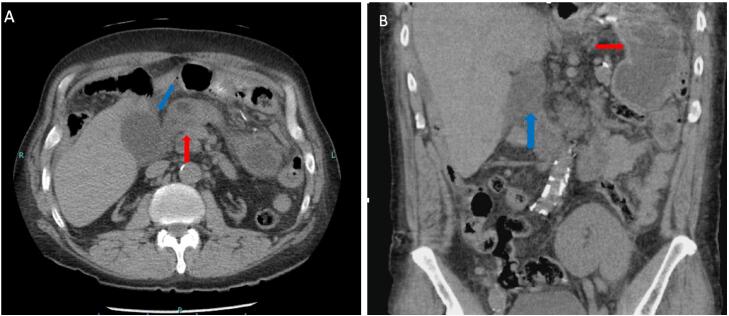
Axial (A) and Coronal (B) CT abdomen/pelvis showing distended gallbladder with wall thickening (blue arrow) and gastric remnant wall thickening with inflammatory stranding adjacent to gallbladder (red arrow). (For interpretation of the references to color in this figure legend, the reader is referred to the web version of this article.)
